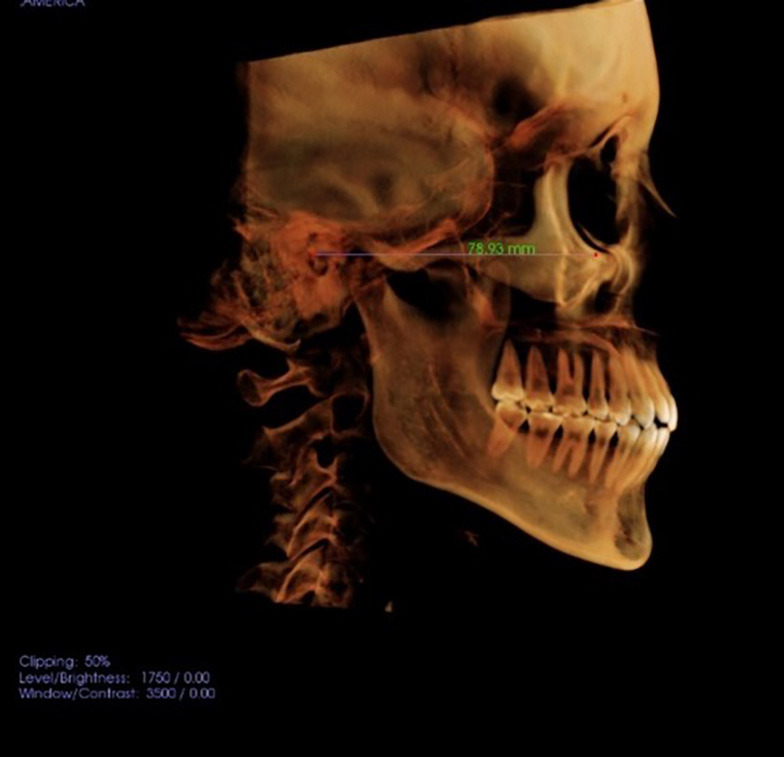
CBCT image of FH-based head alignment.
